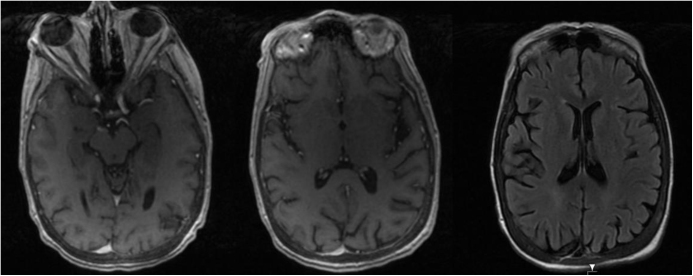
MRI brain with and without contrast – T1 weighted FSE images.
